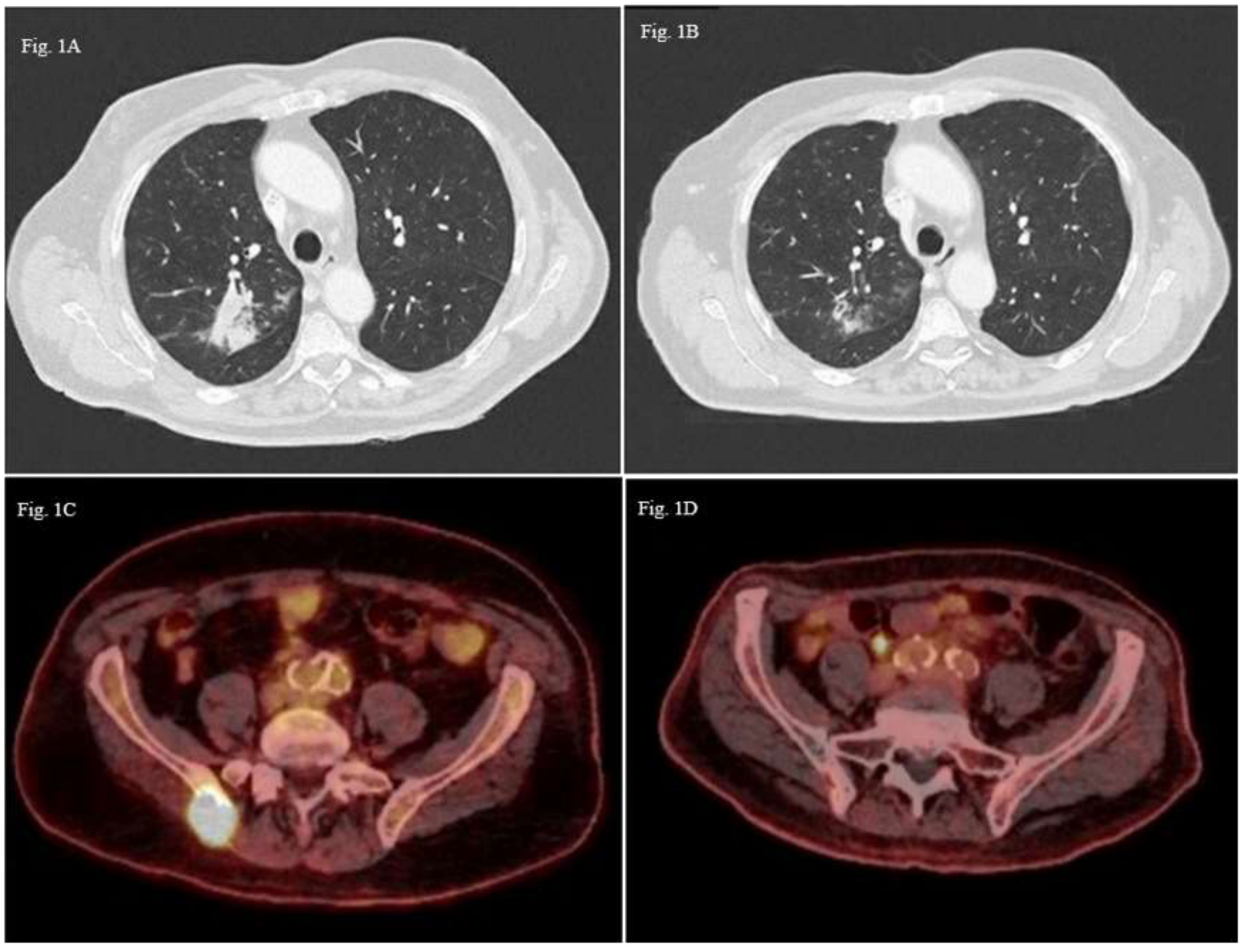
Thoracic CT-scan images axial sections (A) Pulmonary lesion in the posterioir right upper lobe (48mm); (B) Partila regression of the pulmonary lesion (22 mm); 18F-FDG PET scan images axial sections (C) Hypermetabolic bone lesion of the right iliac spine; (D) Partial metabolic response of the bone lesion.
